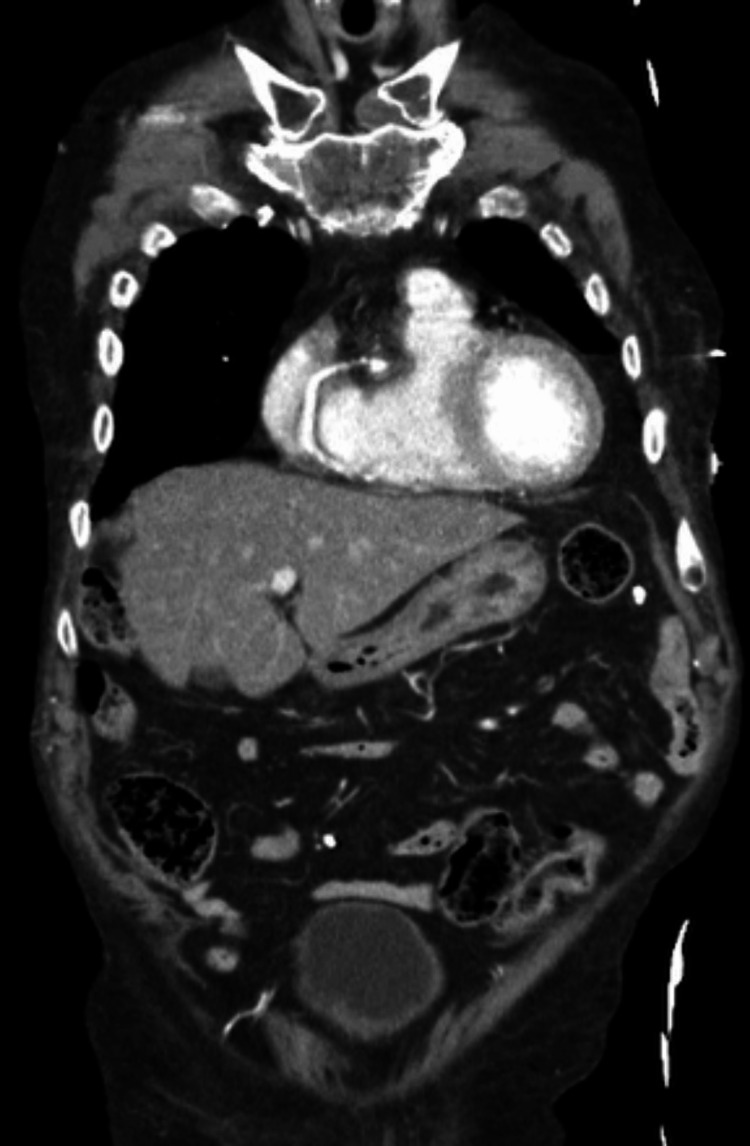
CT abdomen/pelvis showing resolved pneumoperitoneum
